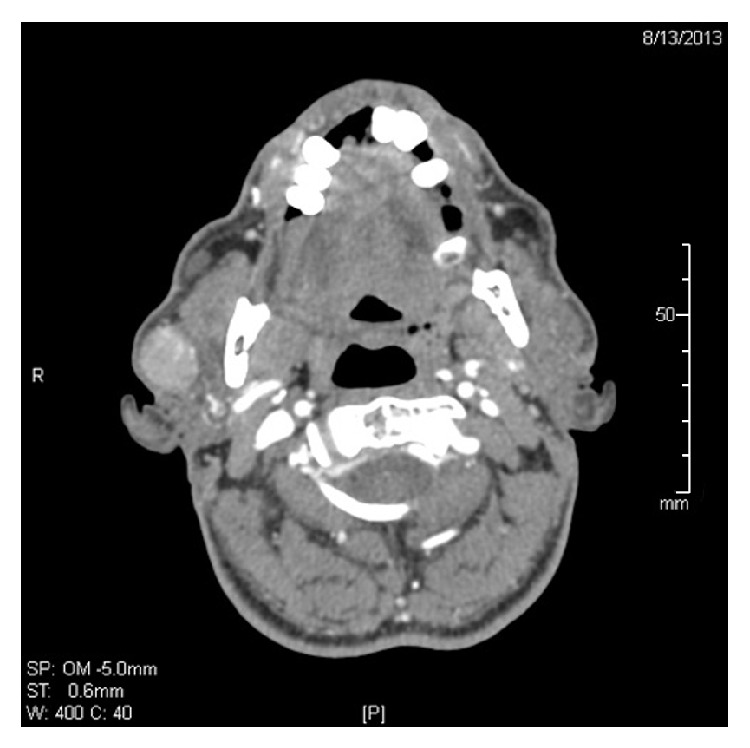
CT neck axial cut, postcontrast, soft tissue window: well-defined oval-shaped mass involving the lobe of the right parotid gland. The lesion shows an increase in density after IV contrast media injection.
